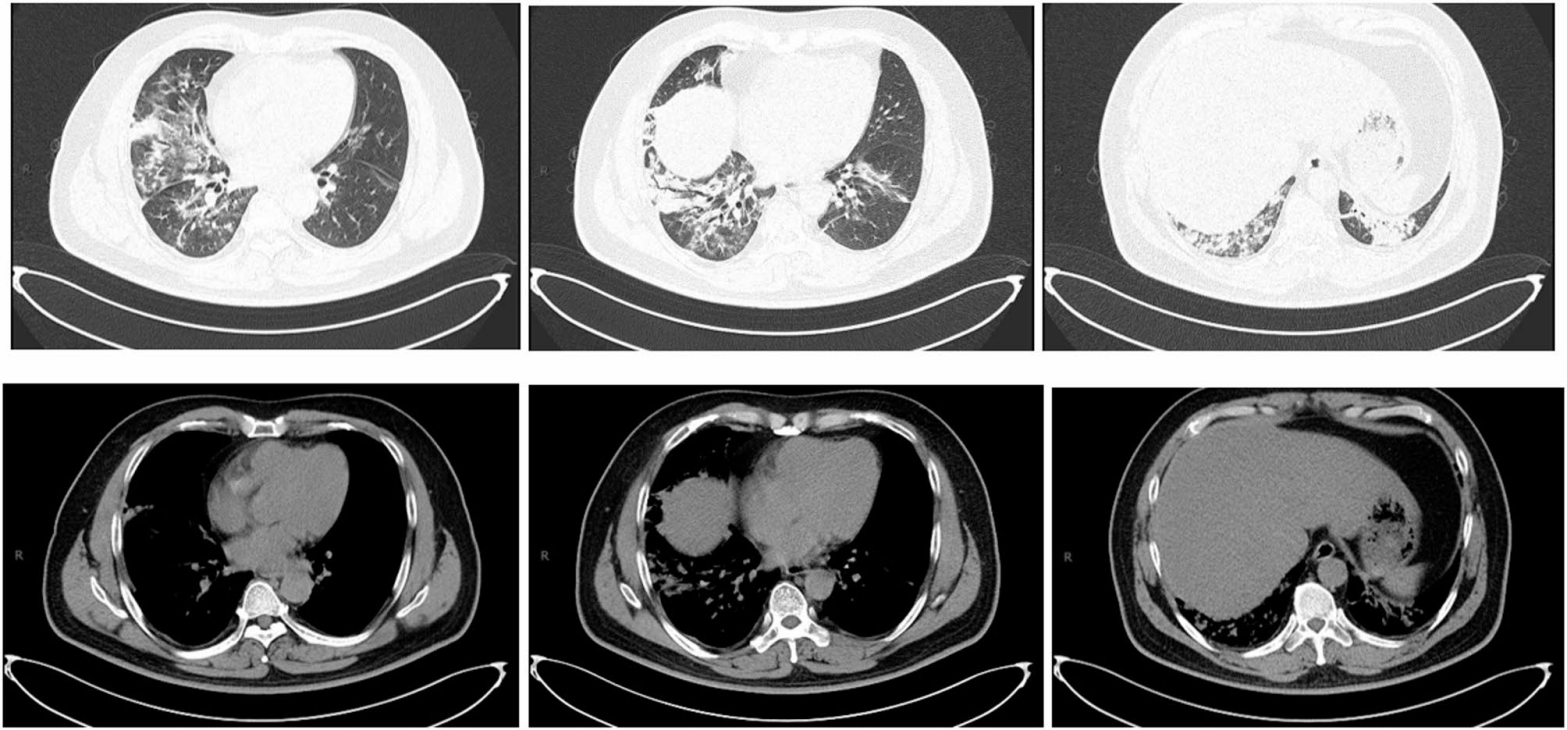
Chest CT scan showed multifocal consolidative opacities on two days before admission to Zhongshan Hospital
